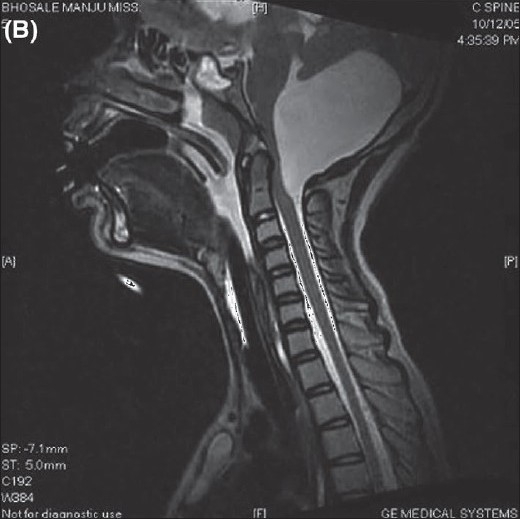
(A) MRI showing large posterior fossa archnoid cyst. (B) MRI showing posterior fossa archnoid cyst causing compression of adjacent structures
